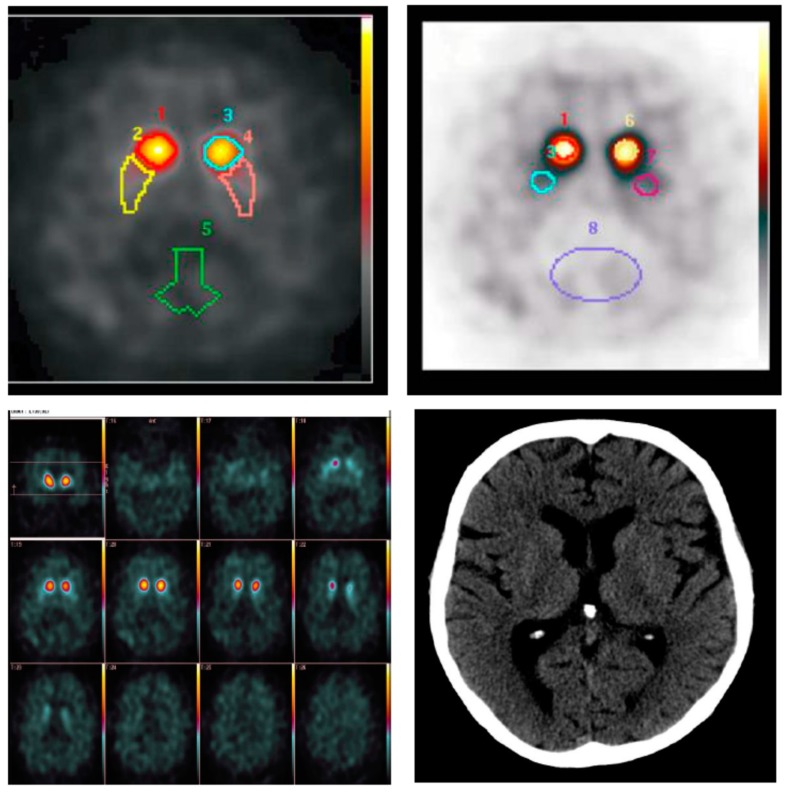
Beta-CIT-Spect and non-contrast computed tomography imaging excerpts from the time of diagnosis.
